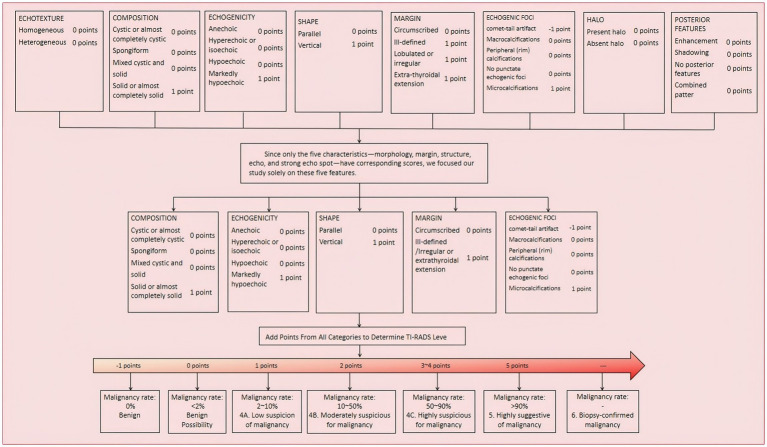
2020 Chinese guidelines for ultrasound risk stratification of thyroid nodules: C-TIRADS.
